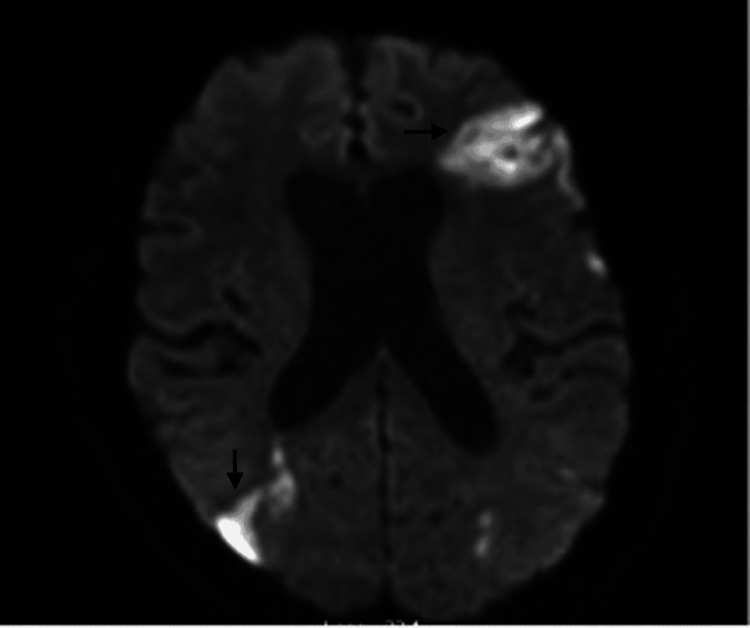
MRI Brain MRI image showing patchy acute infarctions within the left frontal lobe and right parietal lobe as indicated by arrows.
